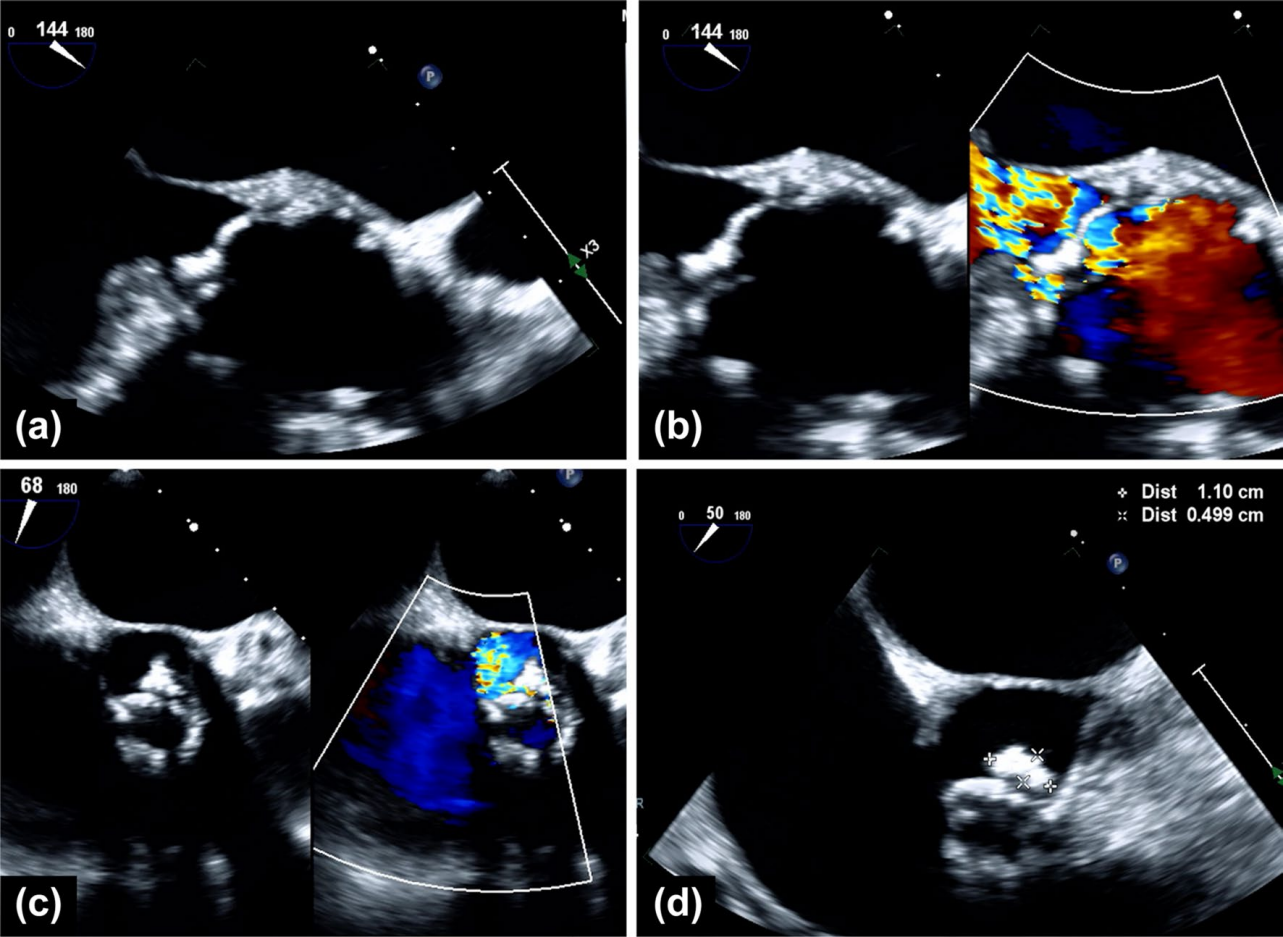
TEE findings. TEE showing prolapse of the thickened left cusp and severe AI in a–c. In addition, an oval structure of approximately 11 mm × 5 mm was attached to the left cusp (d). *TEE* transesophageal echocardiology, *AI* aortic insufficiency
